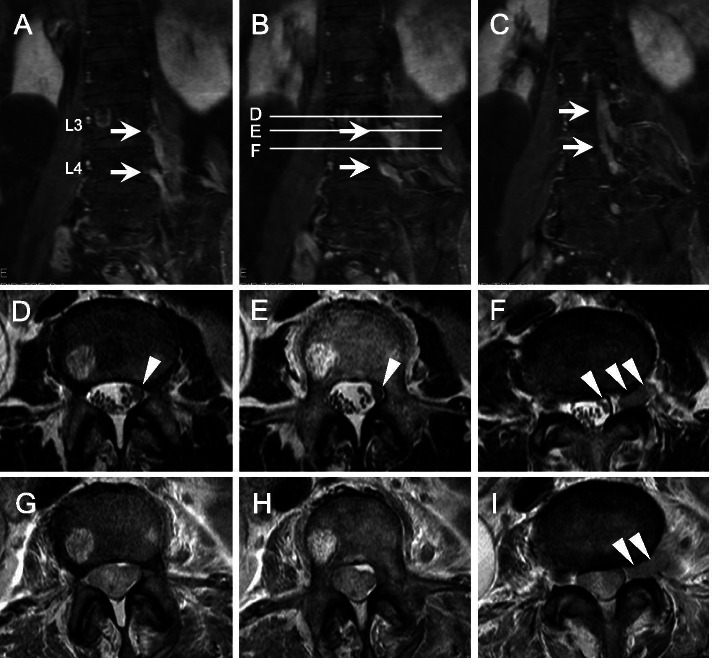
MR image of the lumbosacral spinal roots and lumbar plexus. Gadolinium-enhanced T1-weighted imaging (T1WI) MRI five months after the onset of symptoms (A-F). On the coronal view, enlarged L3 and L4 spinal nerves and lumbar plexus with marked contrast enhancement (arrows) are visible on the left side (A-C). D-F show axial T2WI slices corresponding to the lines on B. Enlarged nerve roots were observed (D-F, arrowheads), and the L4 nerve root filled the intervertebral foramen (F). In the spinal canal, the affected nerves and normal-sized nerves coexisted. G-I show T2WI slices corresponding to the levels in D-F at ten months after onset. G-I show the enlarged cauda equina that was tangled and filled the spinal canal, and I shows the enlarged L4 nerve root (arrowheads)
